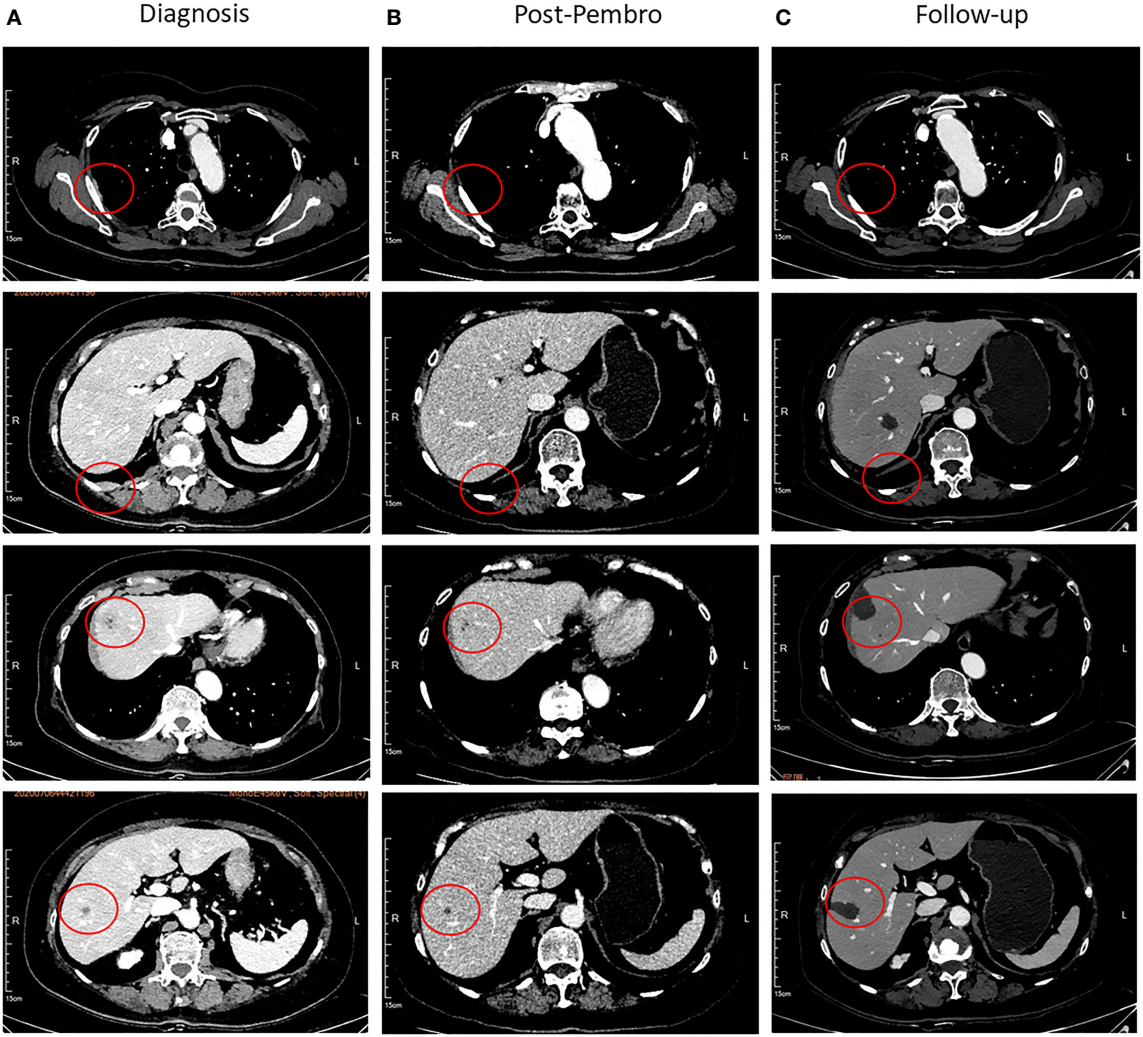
Representative images of the CT scan. (A) The pleura and liver lesions at diagnosis, lesion has been marked with red circles. (B) Disappearance of pleura lesions and shrinkage of liver lesions after four cycles of chemotherapy combined with pembrolizumab. (C) Follow-up after radiofrequency ablation.
